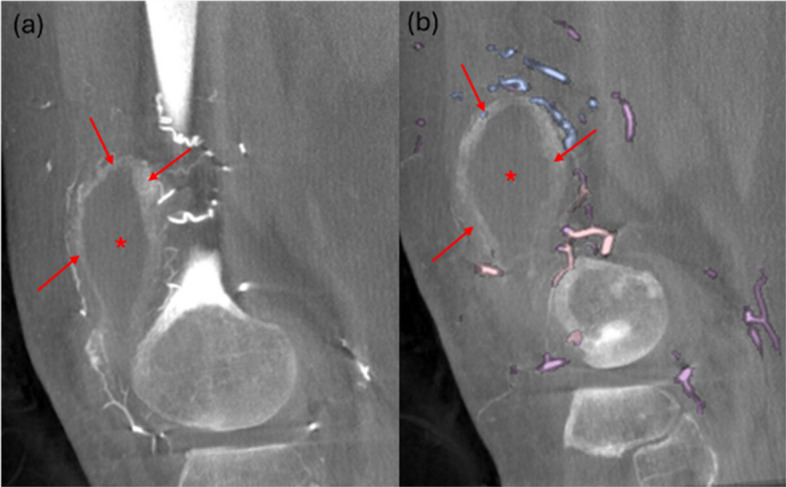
a Sagittal view from contrasted CBCT with injection from Superficial Femoral Artery (SFA) demonstrating joint effusion (*), synovial thickening (red arrows) and hyperemia. b Sagittal view with Embo ASSIST AI vessel segmentation overlay highlighting vessels supplying the pathological area. Arrows indicate synovial thickening, and * denotes joint effusion
